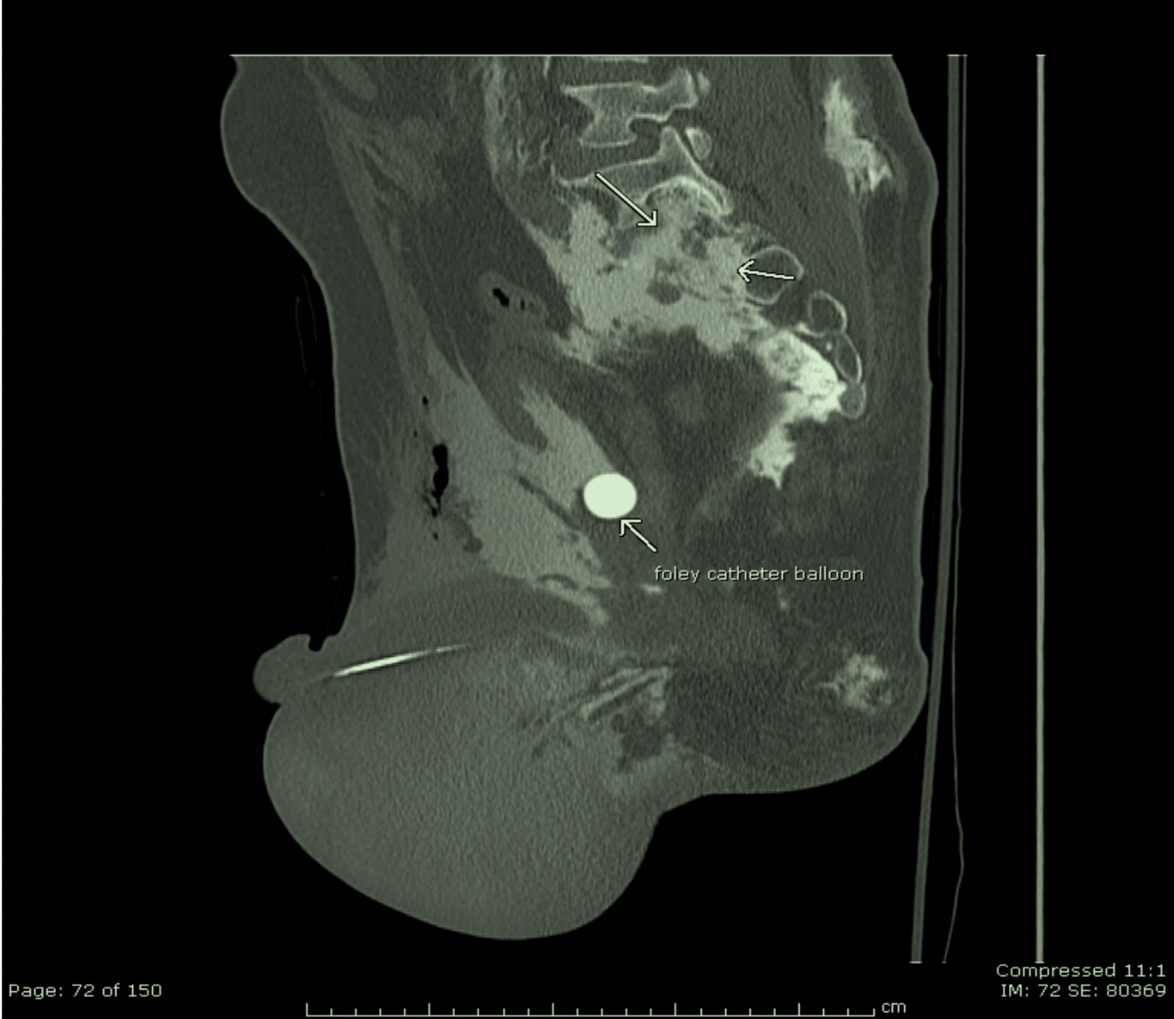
CT of the thoracolumbar spine with coronal and sagittal reconstructions CT: computed tomography
